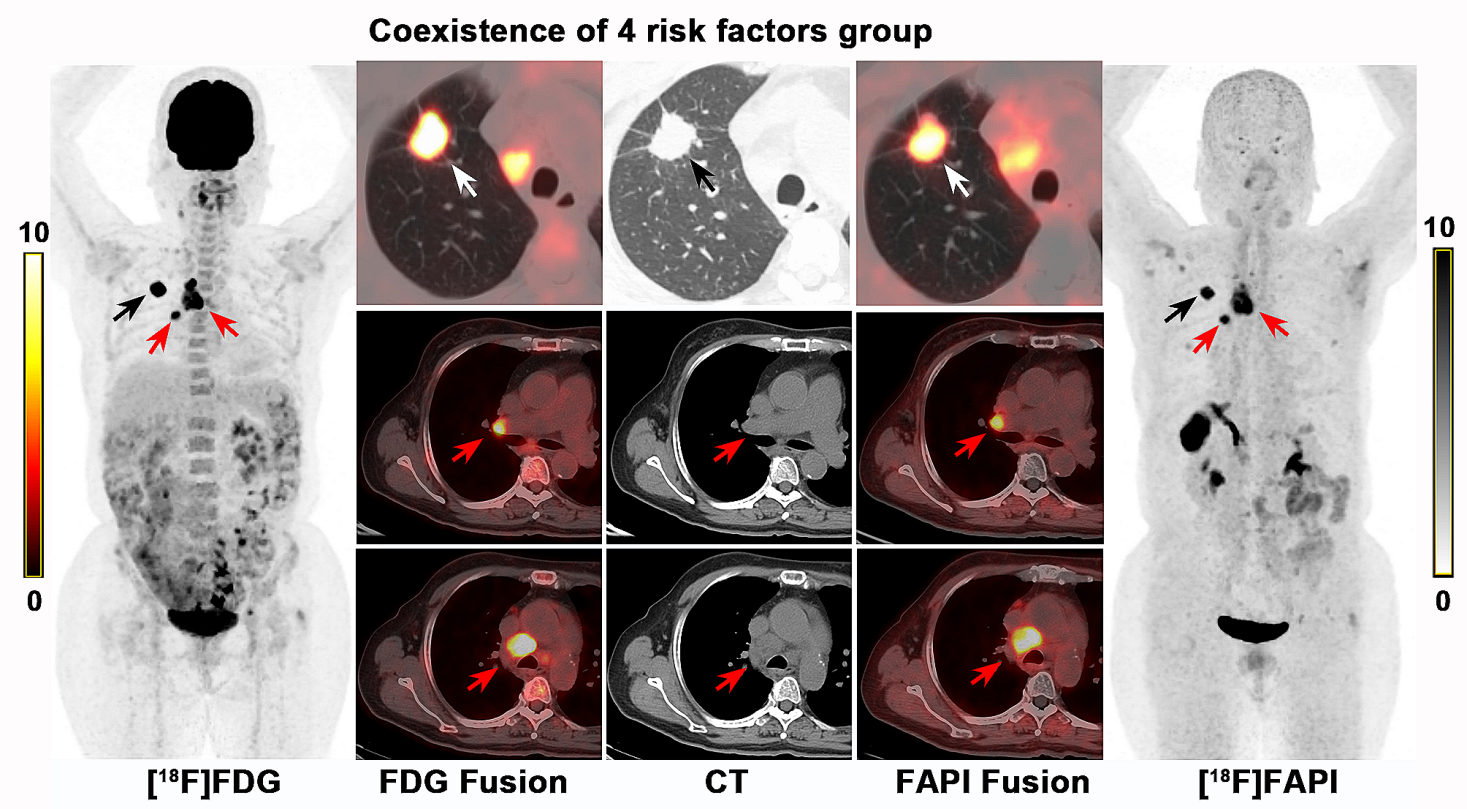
A 65-year-old woman was diagnosed as invasive adenocarcinoma of right upper lung (black or white arrow). The right hilar (10R) and mediastinal (4R) lymph nodes (red arrows) were all high-risk regional lymph nodes with a diameter greater than 1.0 cm (1.1–2.6 cm) and no CHA. These lymph nodes showed high [^18^F]FDG (SUV_max_=10.9–21.3) and [^18^F]FAPI (SUV_max_ =10.0-12.5) uptake. Surgical pathology subsequently confirmed these lymph nodes as metastatic
